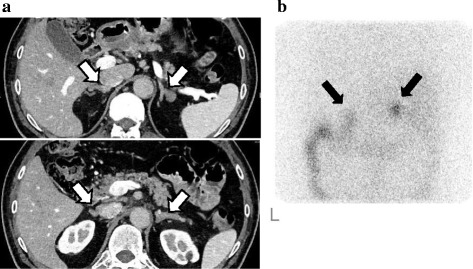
Clinical images. Abdominal CT scan shows nodular enlargement of bilateral adrenal glands (arrows) (a).^131^ I-Adosterol scintigraphy shows increased uptake of radioactivity to bilateral adrenal glands (b)
